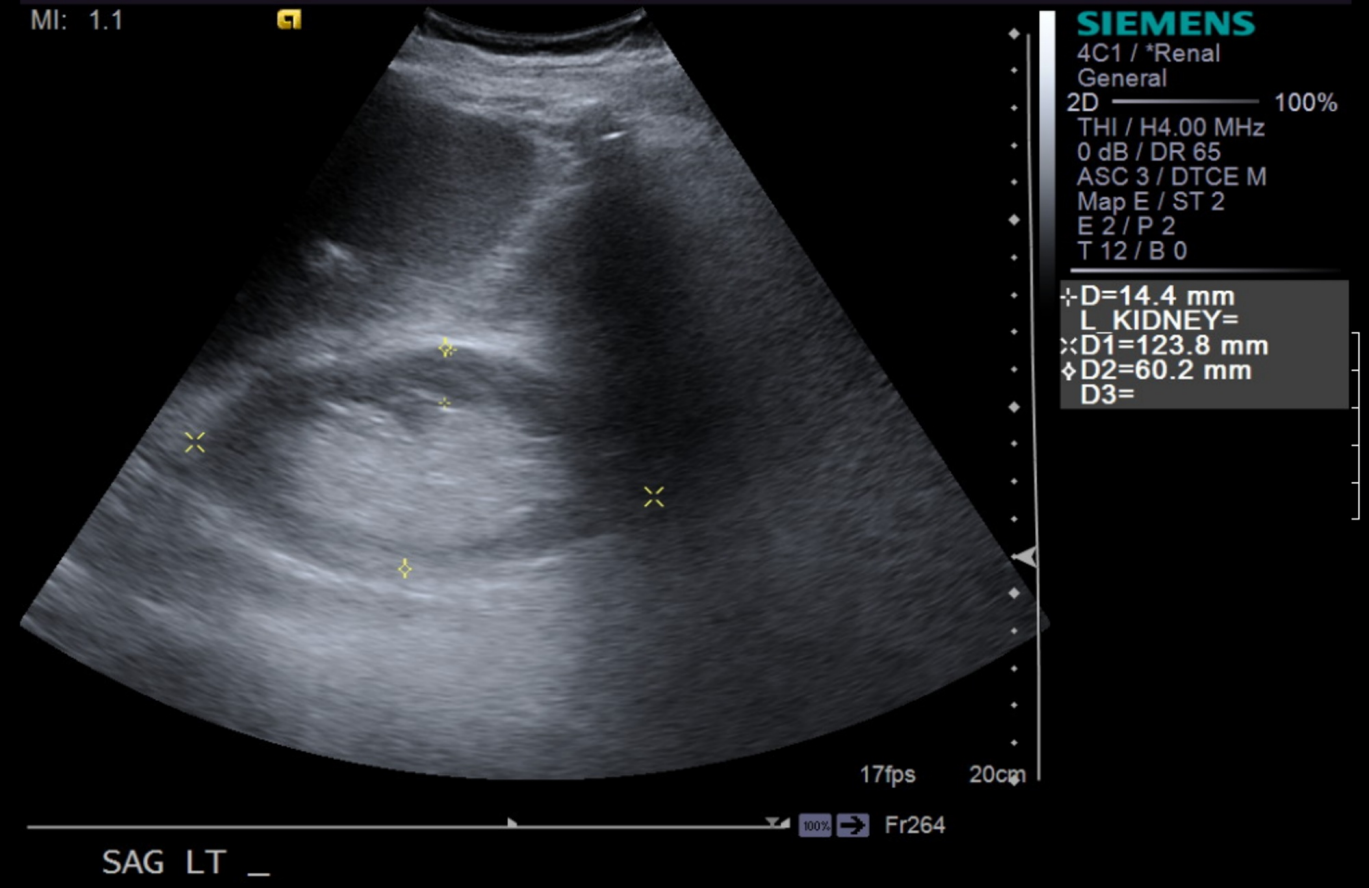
Ultrasound image of the left kidney (yellow asterisk)
